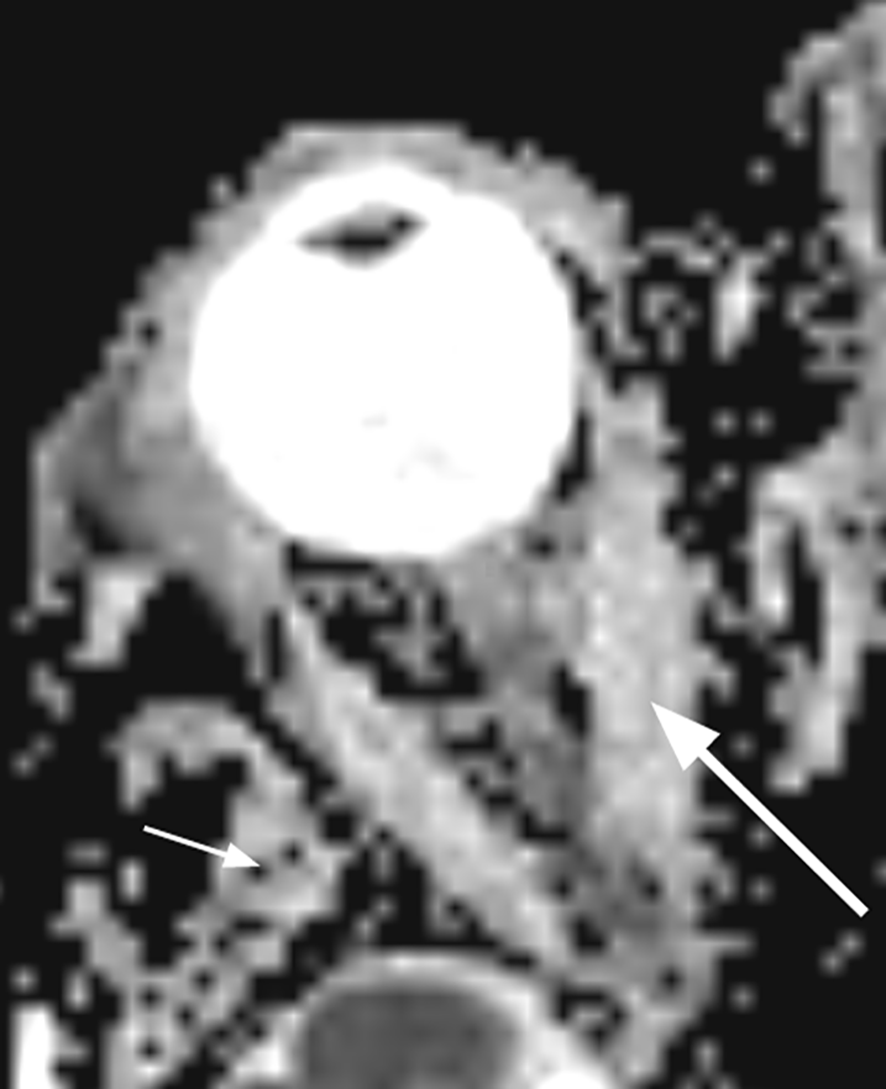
DWI image. To standardize measurements across scanners, a ratio between the ADC of a ROI in the temporalis muscle and the ADC of ROIs in the rectus muscles can be calculated. Small arrow, temporalis muscle; large arrow, medial rectus muscle. DWI, diffusion-weighted imaging; ADC, apparent diffusion coefficient; ROI, region of interest.
